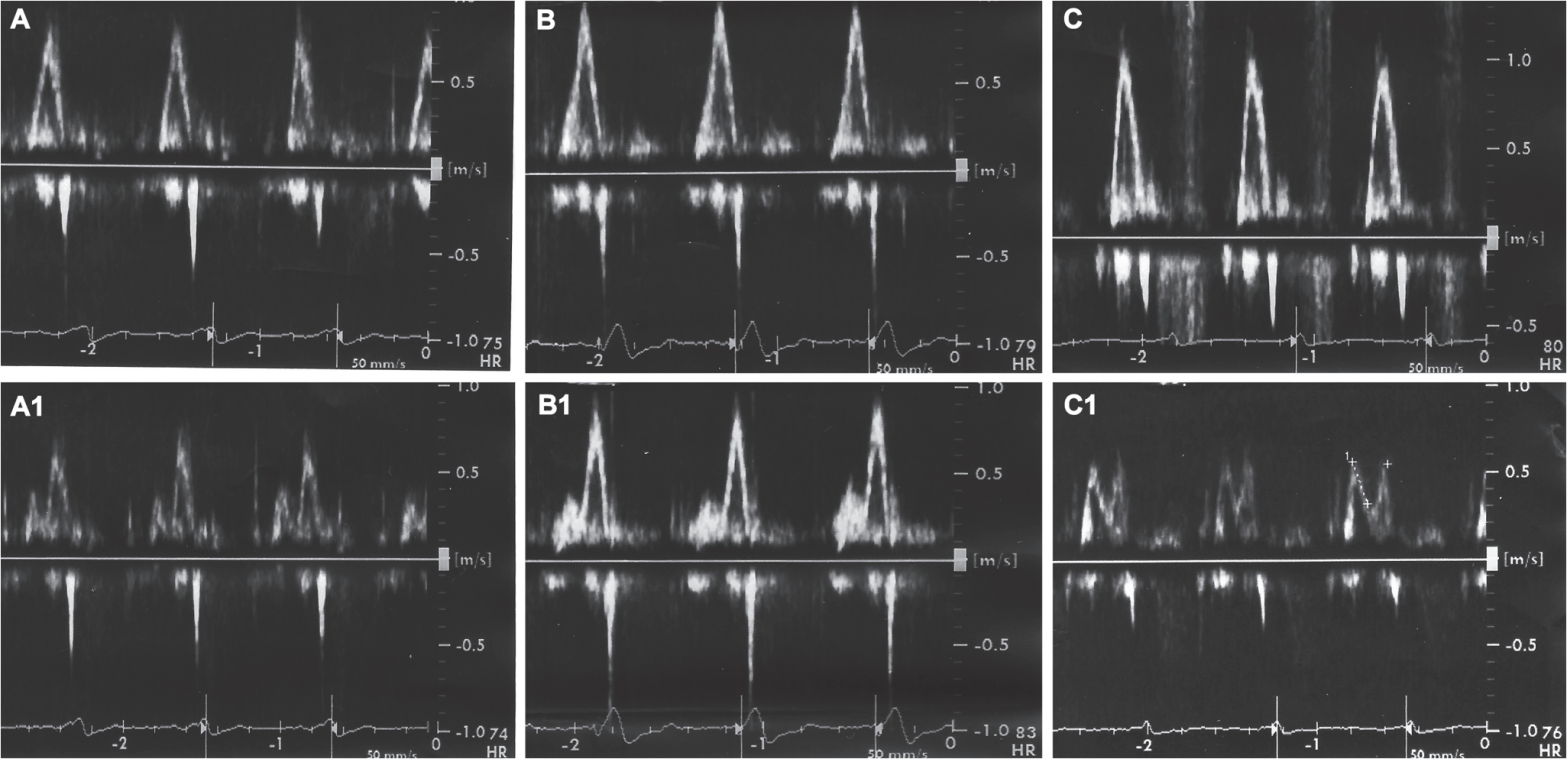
AVI reduction resolves E/A fusion. Transmitral PW Doppler recordings of three exemplary patients with E/A fusion under the nominal AV-sense intervals (125 ms in A, B, C). With AV-sense interval reduction to 75–100 ms (A1, B1, C1) the fusions resolve and E and A waves separate, indicating an improved transmitral inflow. The presented patients are representative for the 19.4% (CI 12.6–26.2%) of patients in sinus rhythm with a too long nominal AV-sense interval.
